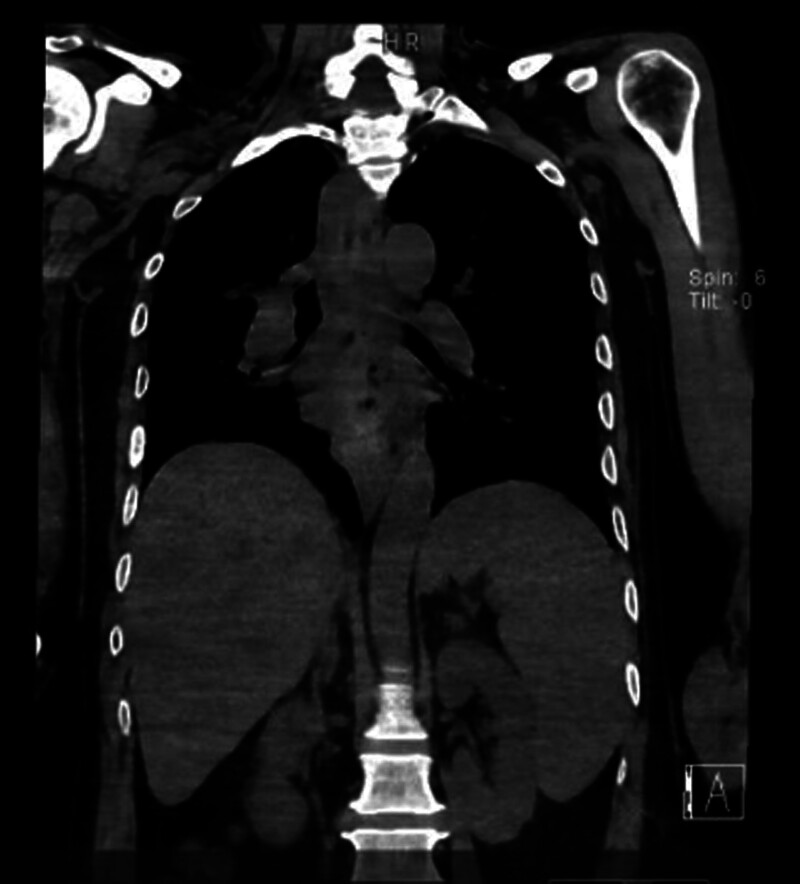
Three-dimensional CT: the esophagus revealed a large amount of retained contents in the middle and upper segments of the esophagus. CT = computed tomography.
